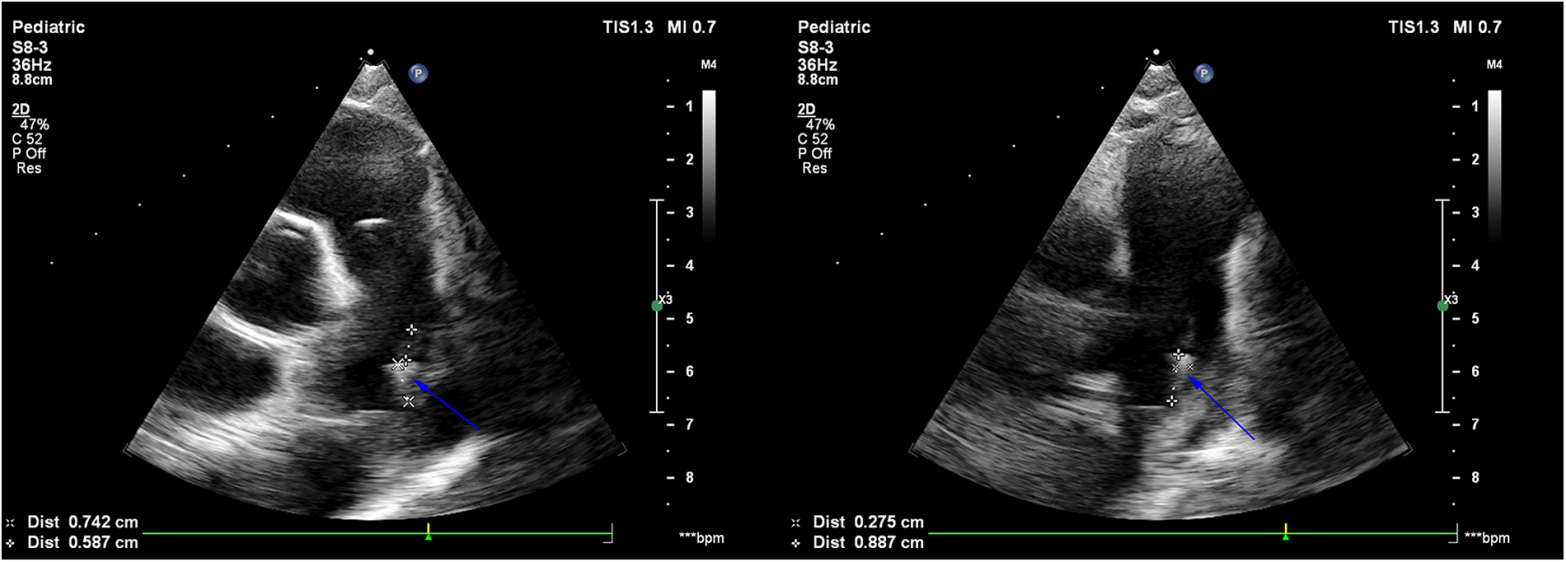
Echocardiogram showed a strip-shaped echo of about 12 mm × 3 mm in size found at the beginning of the left pulmonary artery on the distal left wall of the pulmonary artery, which was fixed in the base, softer in the middle and lower parts, and oscillating obviously, which was considered to be infective vegetation (blue arrow).
